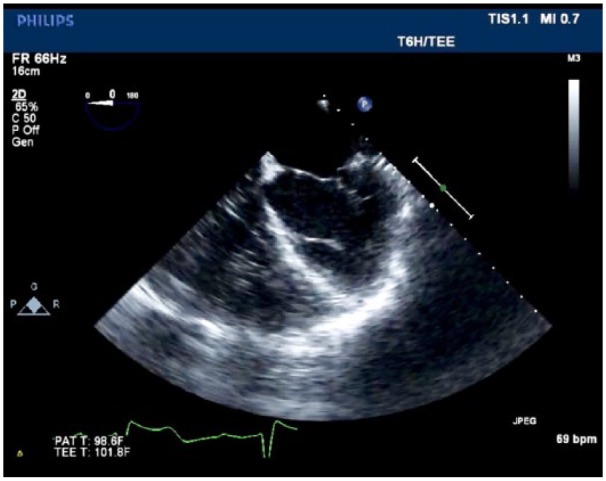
TEE (transesophageal echocardiogram) with bubble study confirming the presence of a persistent left superior vena cava.
